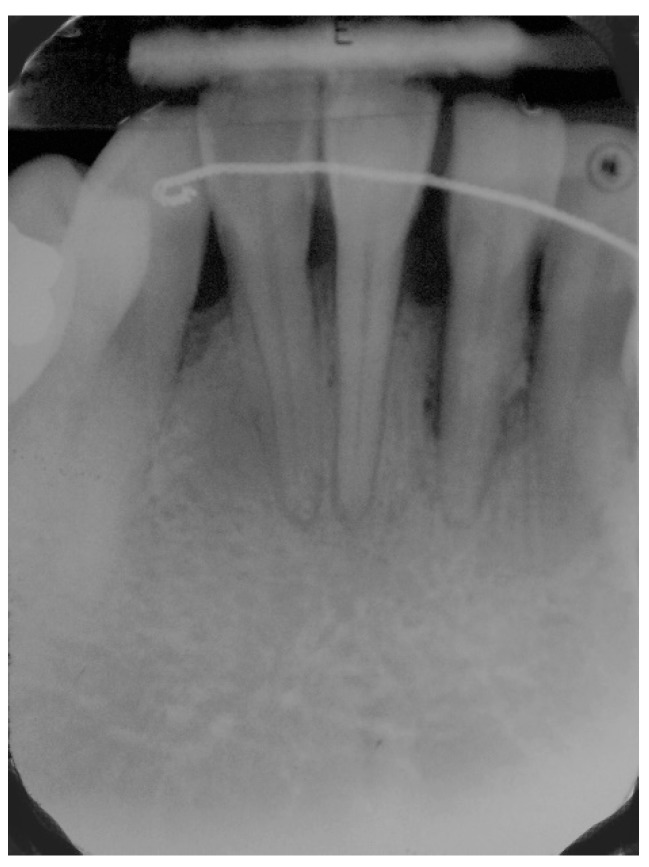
Pre-operative radiograph of lower anterior area showing reduced bone support, with mainly horizontal bone loss.
